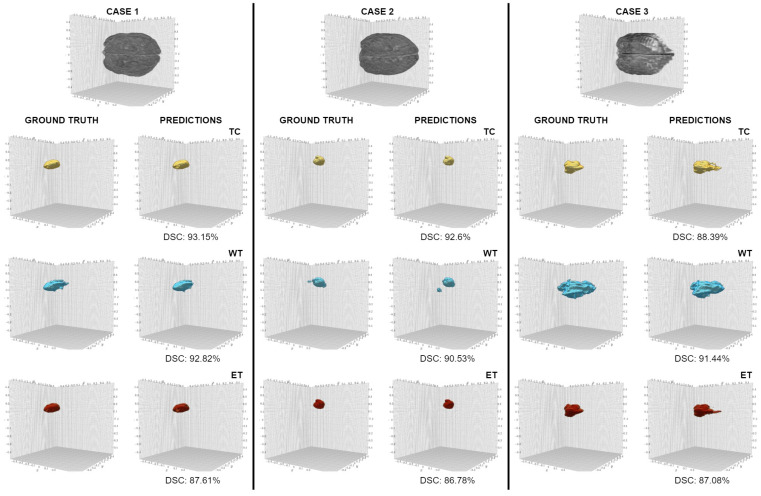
Brain tumour subregion segmentation in three randomly selected MRI cases from the test UCSF-PDGM dataset. The tumour subcategories: tumour core (TC), whole tumour (WT), and enhancing tumour are highlighted in yellow, blue, and red, respectively.
